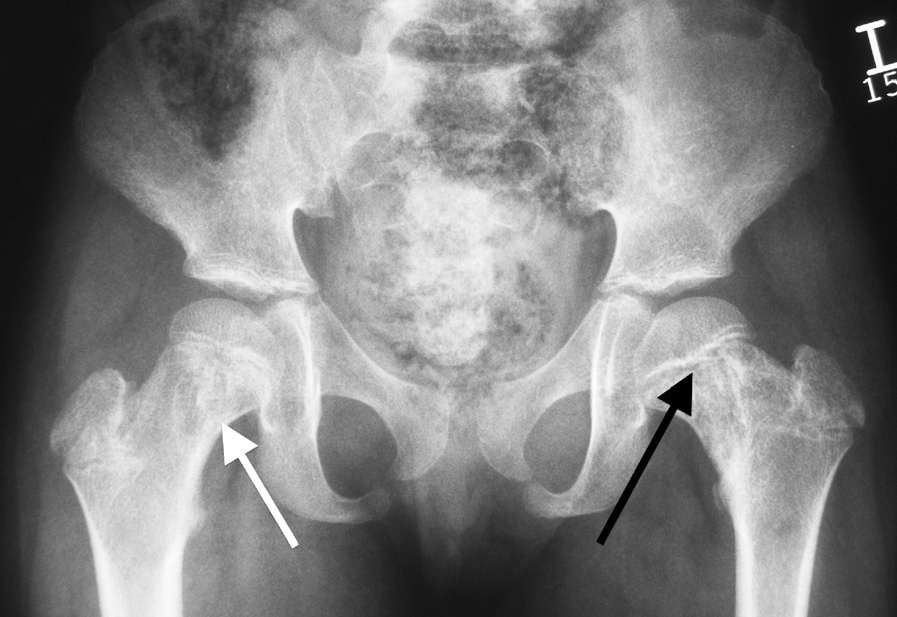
Pelvis radiograph of UPN1 at referral for BMT. There is prominent sclerosis and lucency in both proximal femoral metaphyses (black arrow) and femoral necks (white arrow). These changes extend into the subtrochanteric regions. The acetabular roofs are horizontal.
